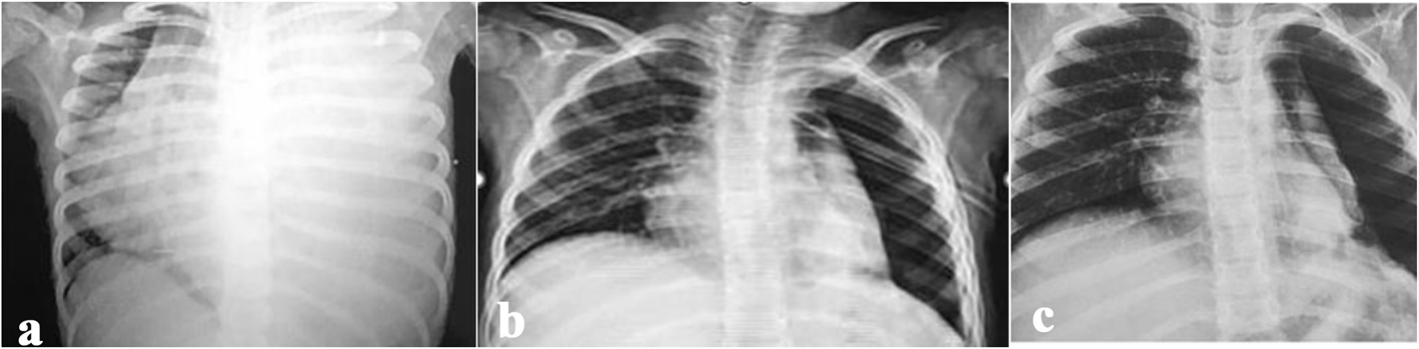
8-year-old female patient presented with grade III respiratory distress and a) chest X-ray showed massive left sided pleural effusion. Nasopharyngeal SARS-CoV-2 RT-PCR was positive. Chest drainage yielded 800 cc pus over 24 h. She also had persistent tachycardia, elevated troponins and echocardiography confirmed diagnosis of carditis and mild pericardial effusion. Two days later, she had worsening respiratory distress and CT chest showed evidence of air-filled cavities and pyo-pneumothorax confirming progression to necrotising pneumonia. She received systemic steroids, intravenous immunoglobulins, in addition to antibiotics. Pleural, blood and sputum cultures were negative. Despite 3 weeks of chest drainage and suction, she had persistent air leak and chest X-ray b) shows a trapped lung, 3 weeks after admission. Air leak resolved after 4 weeks and chest drain was removed, however, c) due to failure of lung expansion and persistent pneumothorax, she was referred for decortication
